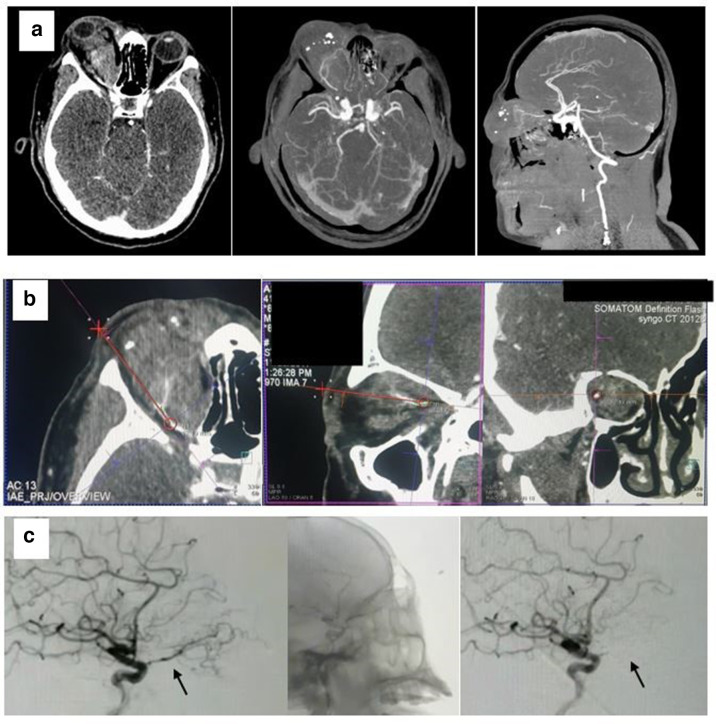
Case 4, hypervascular tumor of the right retroorbital region. (a) CTA of the carotid showing the hypervascular mass of the right retroorbital region supplied by branches of the right ophthalmic artery. (b) CBCT-A in axial, sagittal, and coronal views demonstrating the projection of the virtual needle targeting the right ophthalmic artery. (c) DSA images showing (left) feeding artery, namely the right ophthalmic artery, (middle) needle insertion to the target vessel, and (right) occlusion of the feeding artery following glue administration. CBCT-A, cone beam computed tomography angiography; CTA, computed tomography angiography; DSA, digital subtraction angiography.
